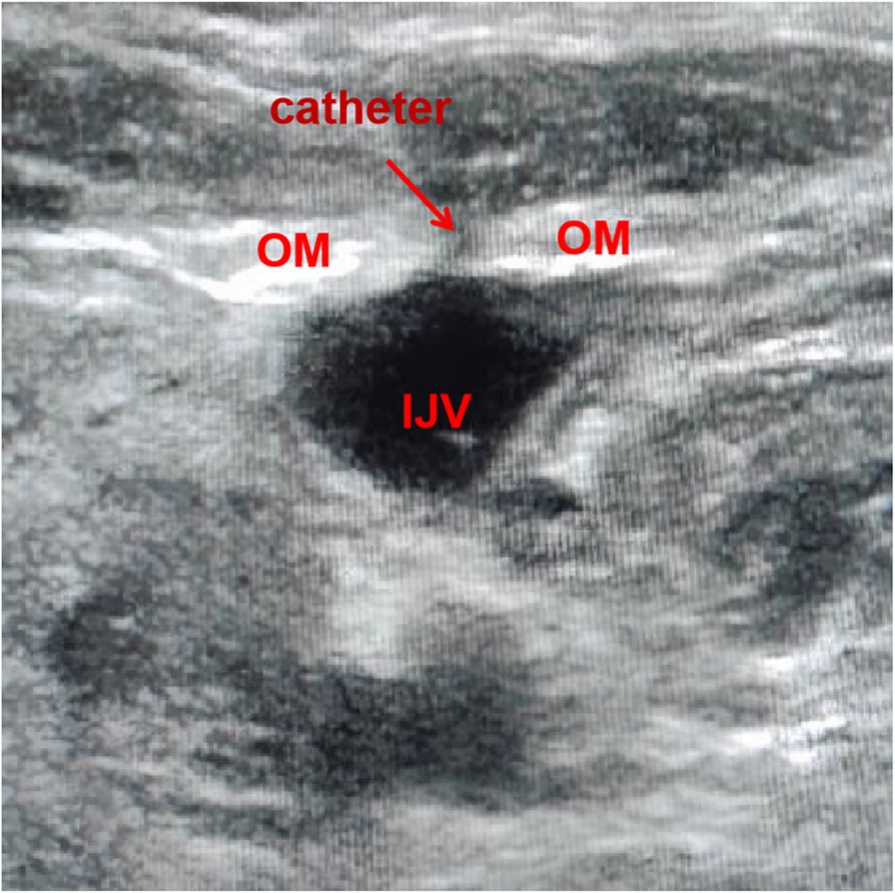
Ultrasound image of catheter traversing the right OMs
